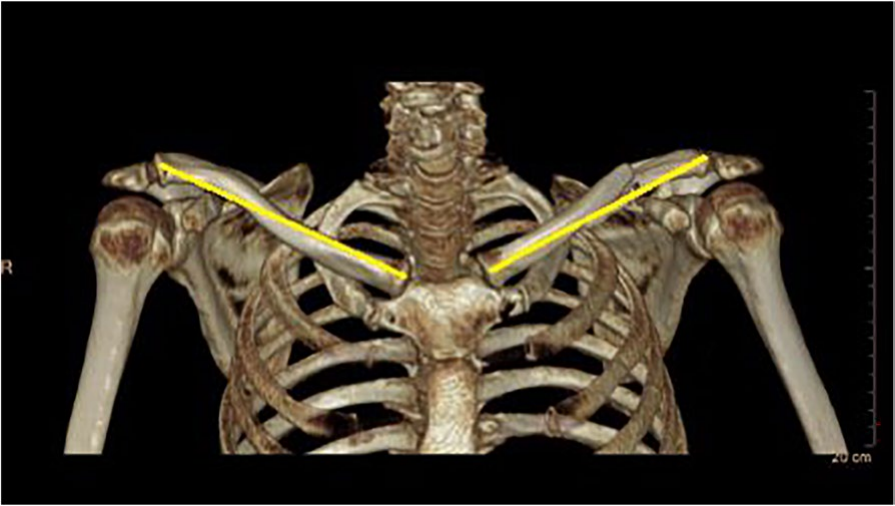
Measurement of clavicle length by computed tomography
